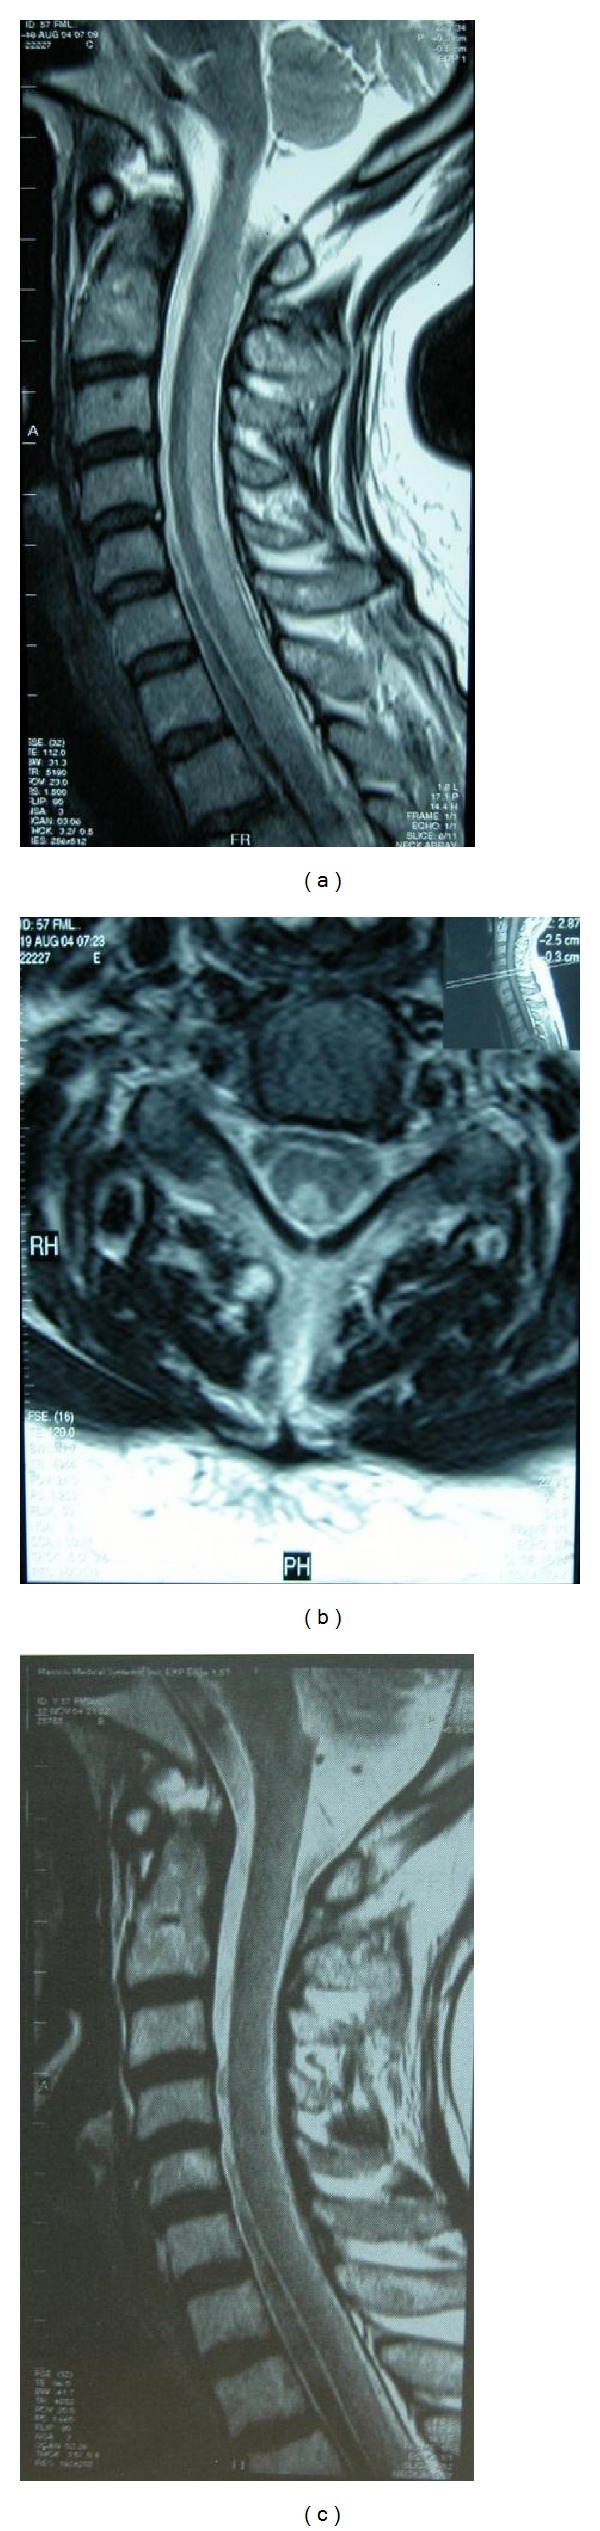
((a), (b)) At the baseline sagittal and axial T2 weighted MRI study, hyperintensity of the posterior columns can be seen in the cervical cord. (c) Posttreatment sagittal T2 magnetic resonance images showed total regression of the MRI abnormalities.
